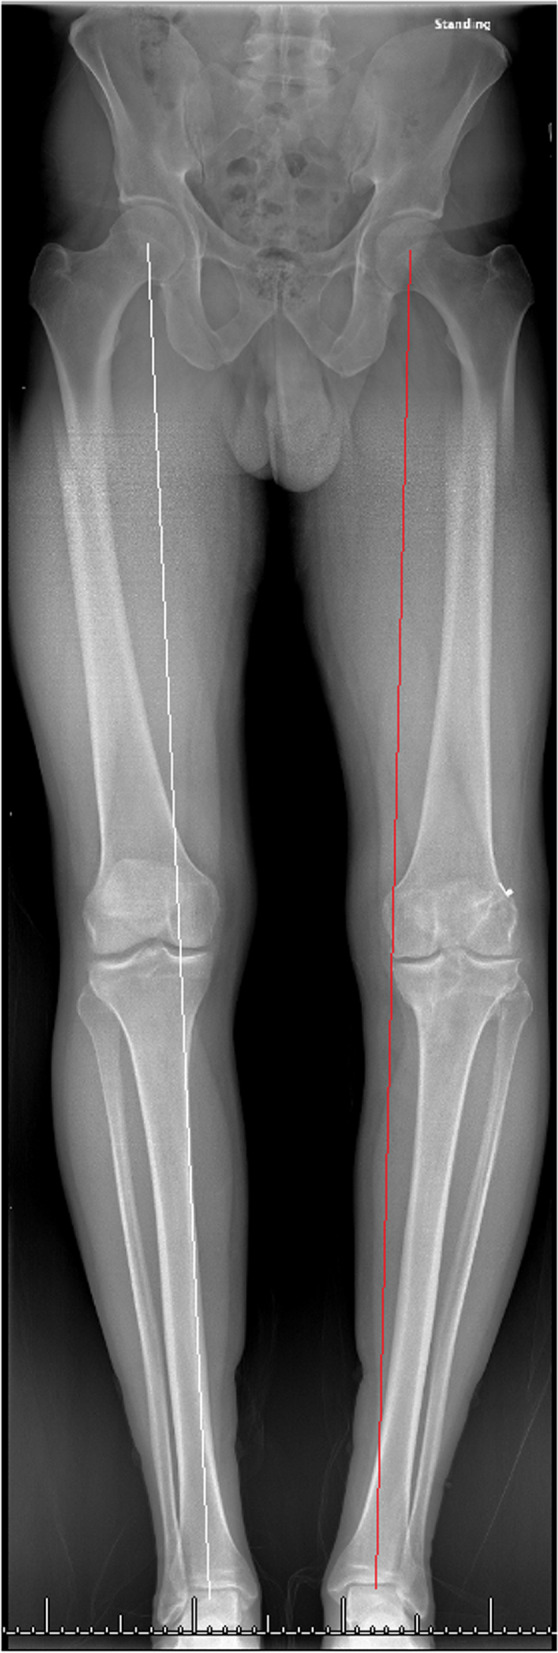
Standing mechanical axis radiograph showing a healed osteotomy without further varus progression
